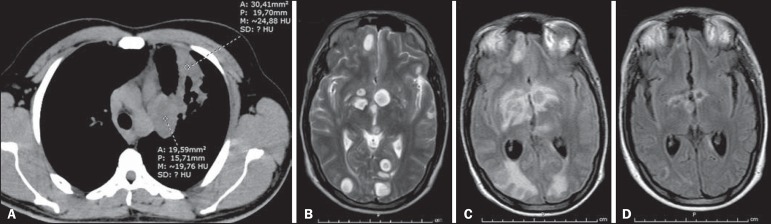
A: Axial computed tomography of the chest showing consolidation
